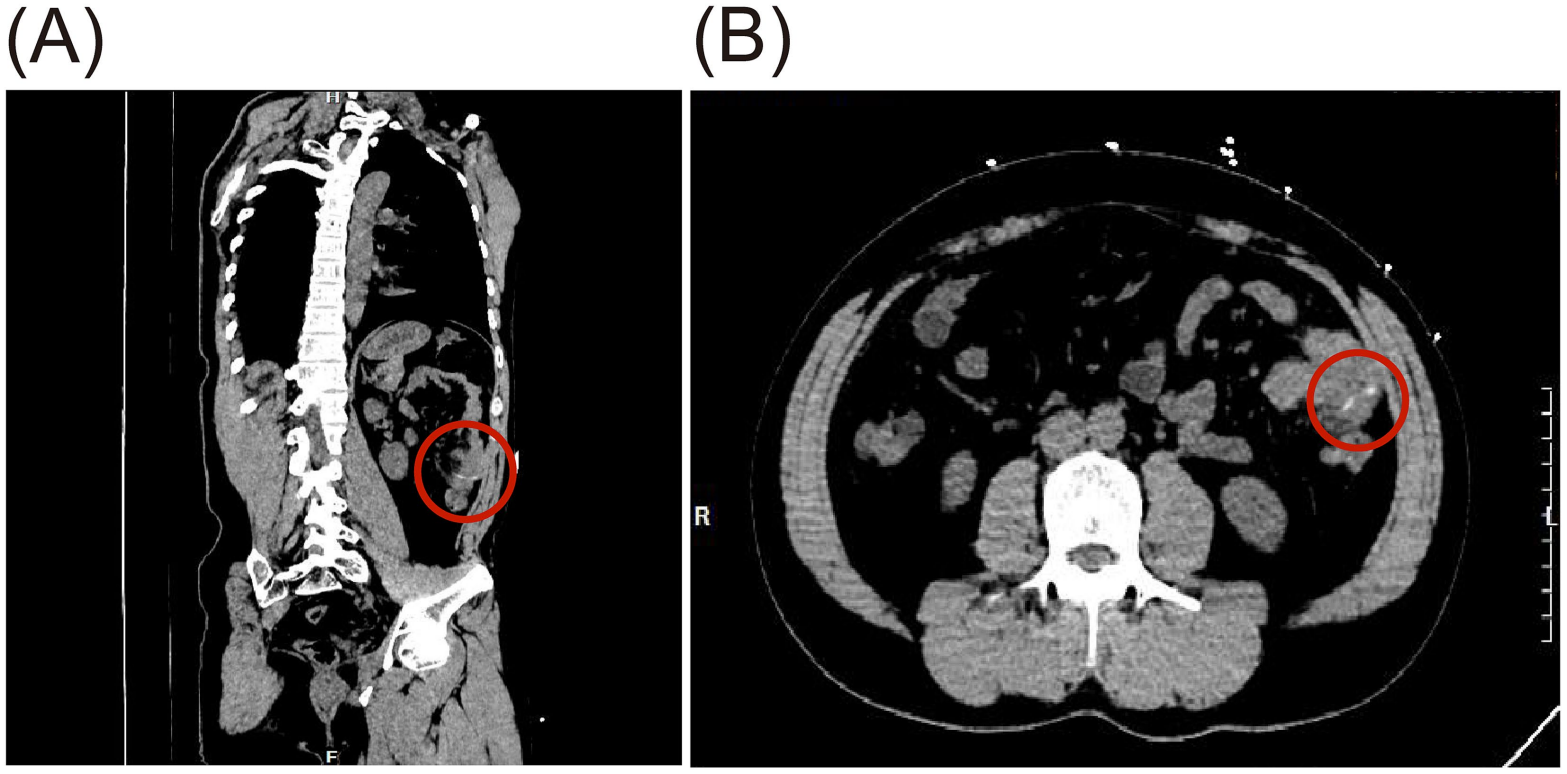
Preoperative CT imaging of small bowel perforation caused by FI, showing a linear hyperdense foreign body about 3 cm long in the small intestine (red circle). (A) Oblique sagittal plane. (B) Coronal plane.
